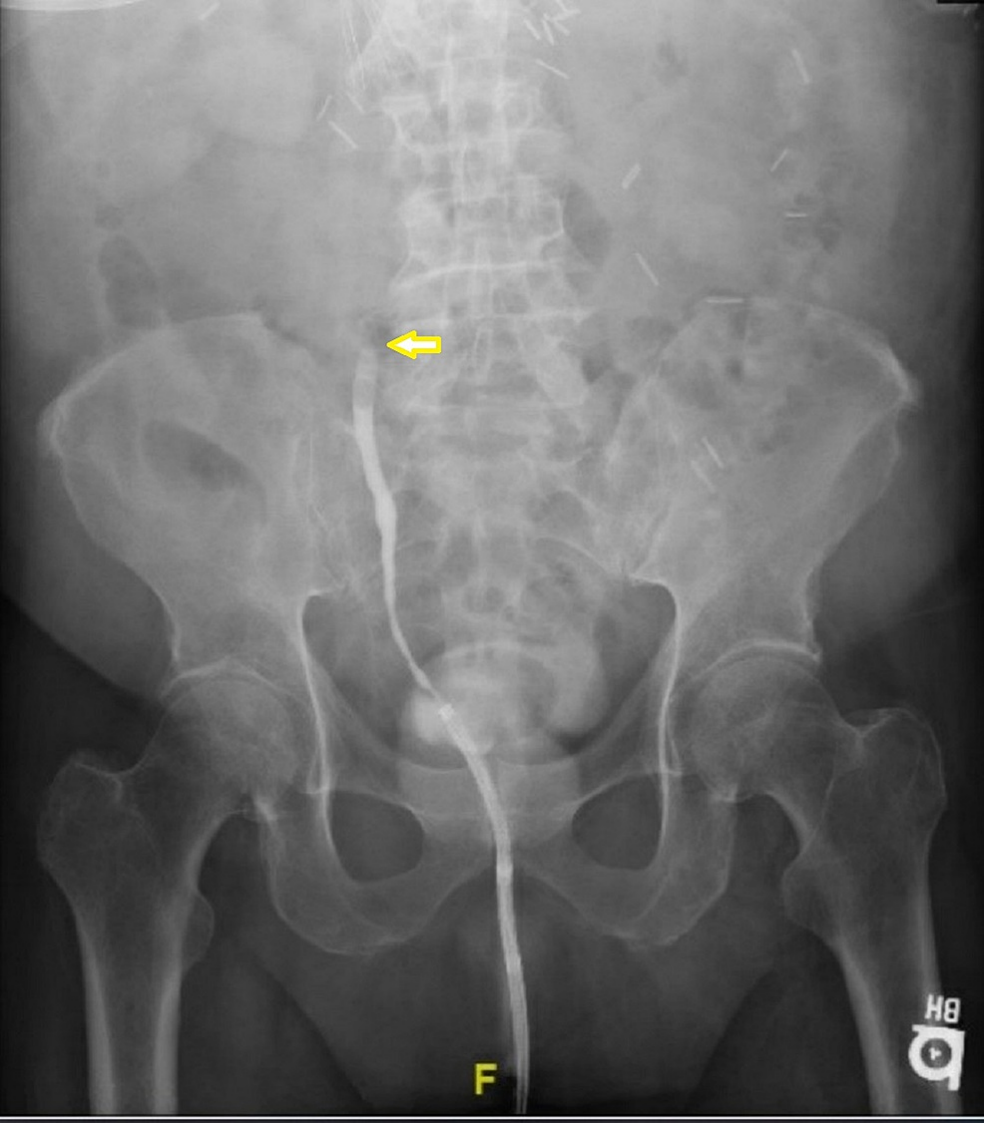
X-ray cystogram showing lack of contrast extension beyond patient's mid ureter (indicated by arrow)
